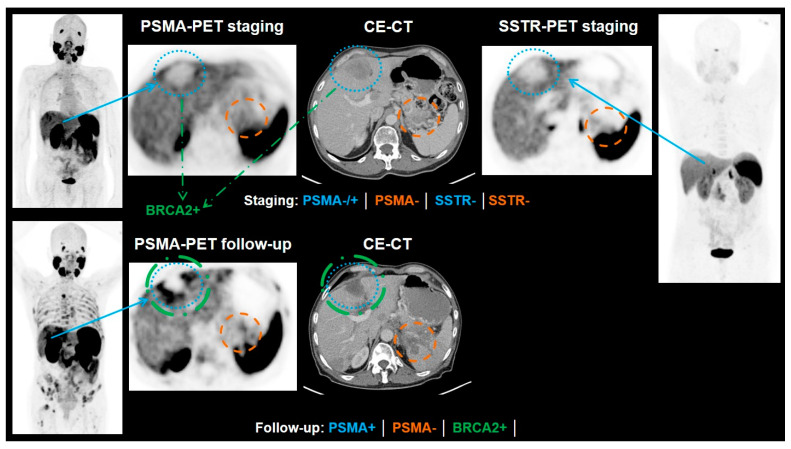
Example of a patient with neuroendocrine differentiated prostate cancer. At the time of the staging PSMA-PET, the patient had already tested positive for neuroendocrine differentiation of the prostate cancer. The PSA value had been below the detection rate. In the PSMA scan, the liver metastases showed only a PSMA expression similar to the liver uptake or only slightly above liver uptake (blue). A metastasis in the pancreas showed no PSMA expression (orange). An additional SSTR-PET was performed where no SSTR expression of the liver metastases (blue), nor was the pancreas metastasis (orange) detectable. After another biopsy of the marked liver metastasis, a BRCA2 mutation could be confirmed (green). The patient underwent chemotherapy with etoposid and cisplatin as well as an immunotherapy with olaparib. In the follow-up PSMA-PET, the patient presented with progressive disease, but the BRCA2-positive metastasis decreased in size (the lesion was additionally treated with Gamma Knife) and showed a higher PSMA expression than before (blue, green). In contrast, the metastasis of the pancreas increased in size and showed still no PSMA expression. CE-CT = contrast-enhancement computed tomography, PSMA = prostate-specific membrane antigen, SSTR = somatostatin receptor.
